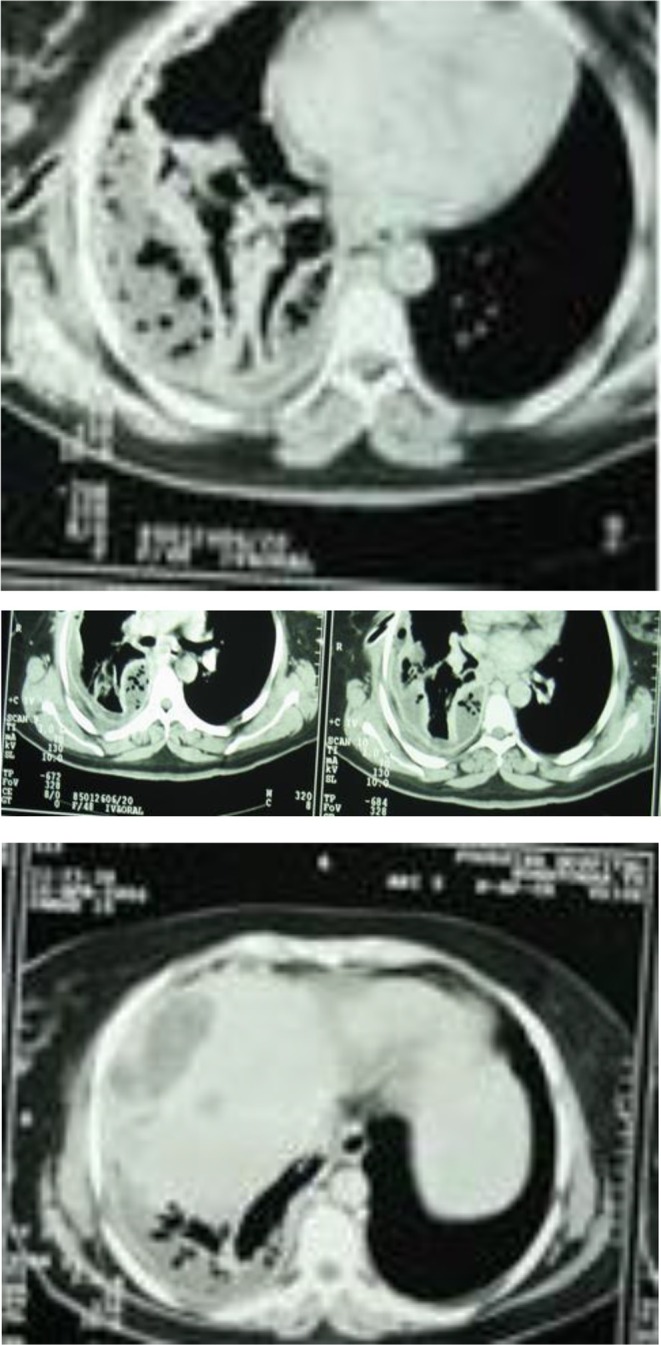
CT‐scan of abdomen, which shows cystic lesions in the liver with pleural effusion.
